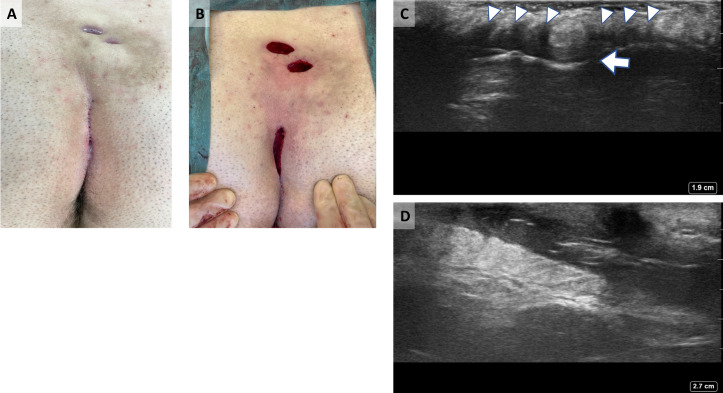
Complex pilonidal Sinus. A) Multiple midline pits and two secondary opening cranially. B)Post modified Lord Millar excision. C) Ultrasonographic view at the midline of natal cleft showing multiple pits (arrowheads) and the pilonidal sinus with hair within (arrow). No craniocaudal extension. D)Ultrasonographic view at the upper part of the natal cleft showing the cranial extension of the pilonidal sinus towards the secondary openings.
